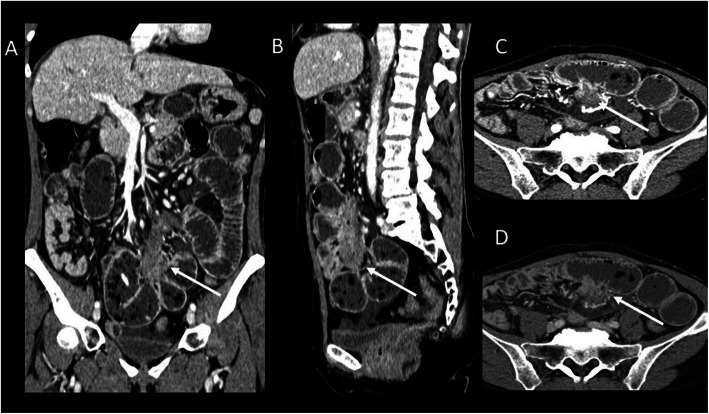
Mesenteric DT. A 54-year-old female patient with a positive history for previous surgery (hystero-annessiectomy) and chronic liver disease. CT images ((a) coronal, (b) sagittal, and (c, d) axial plane) show a poorly defined border lesion (arrow) with radiating spicules extending into the adjacent mesenteric fat and infiltrating the small bowel causing small bowel obstruction. Axial arterial phase (c) and portal phase (d) show progressive contrast enhancement of the lesion
